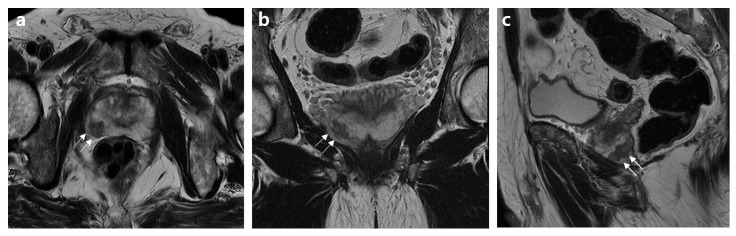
MR images of a 77-year-old patient with an extensive extraprostatic extension (EPE). (a) Axial, (b) coronal, and (c) sagittal T2-weighted MR images show a frank breach of the prostate capsule associated with a right peripheral zone mid-posterior lesion (arrows). The basic clinical findings included a serum PSA of 14.7 ng/mL, a prostate volume of 33 mL, a positive biopsy core of 38%, clinical stage of T3a, biopsy grade 4, and high D’Amico risk. The PI-RADS v2.1 scores and Mehralivand EPE grades on multiparametric MRI were 5 and 3, respectively, according to both radiologists.
